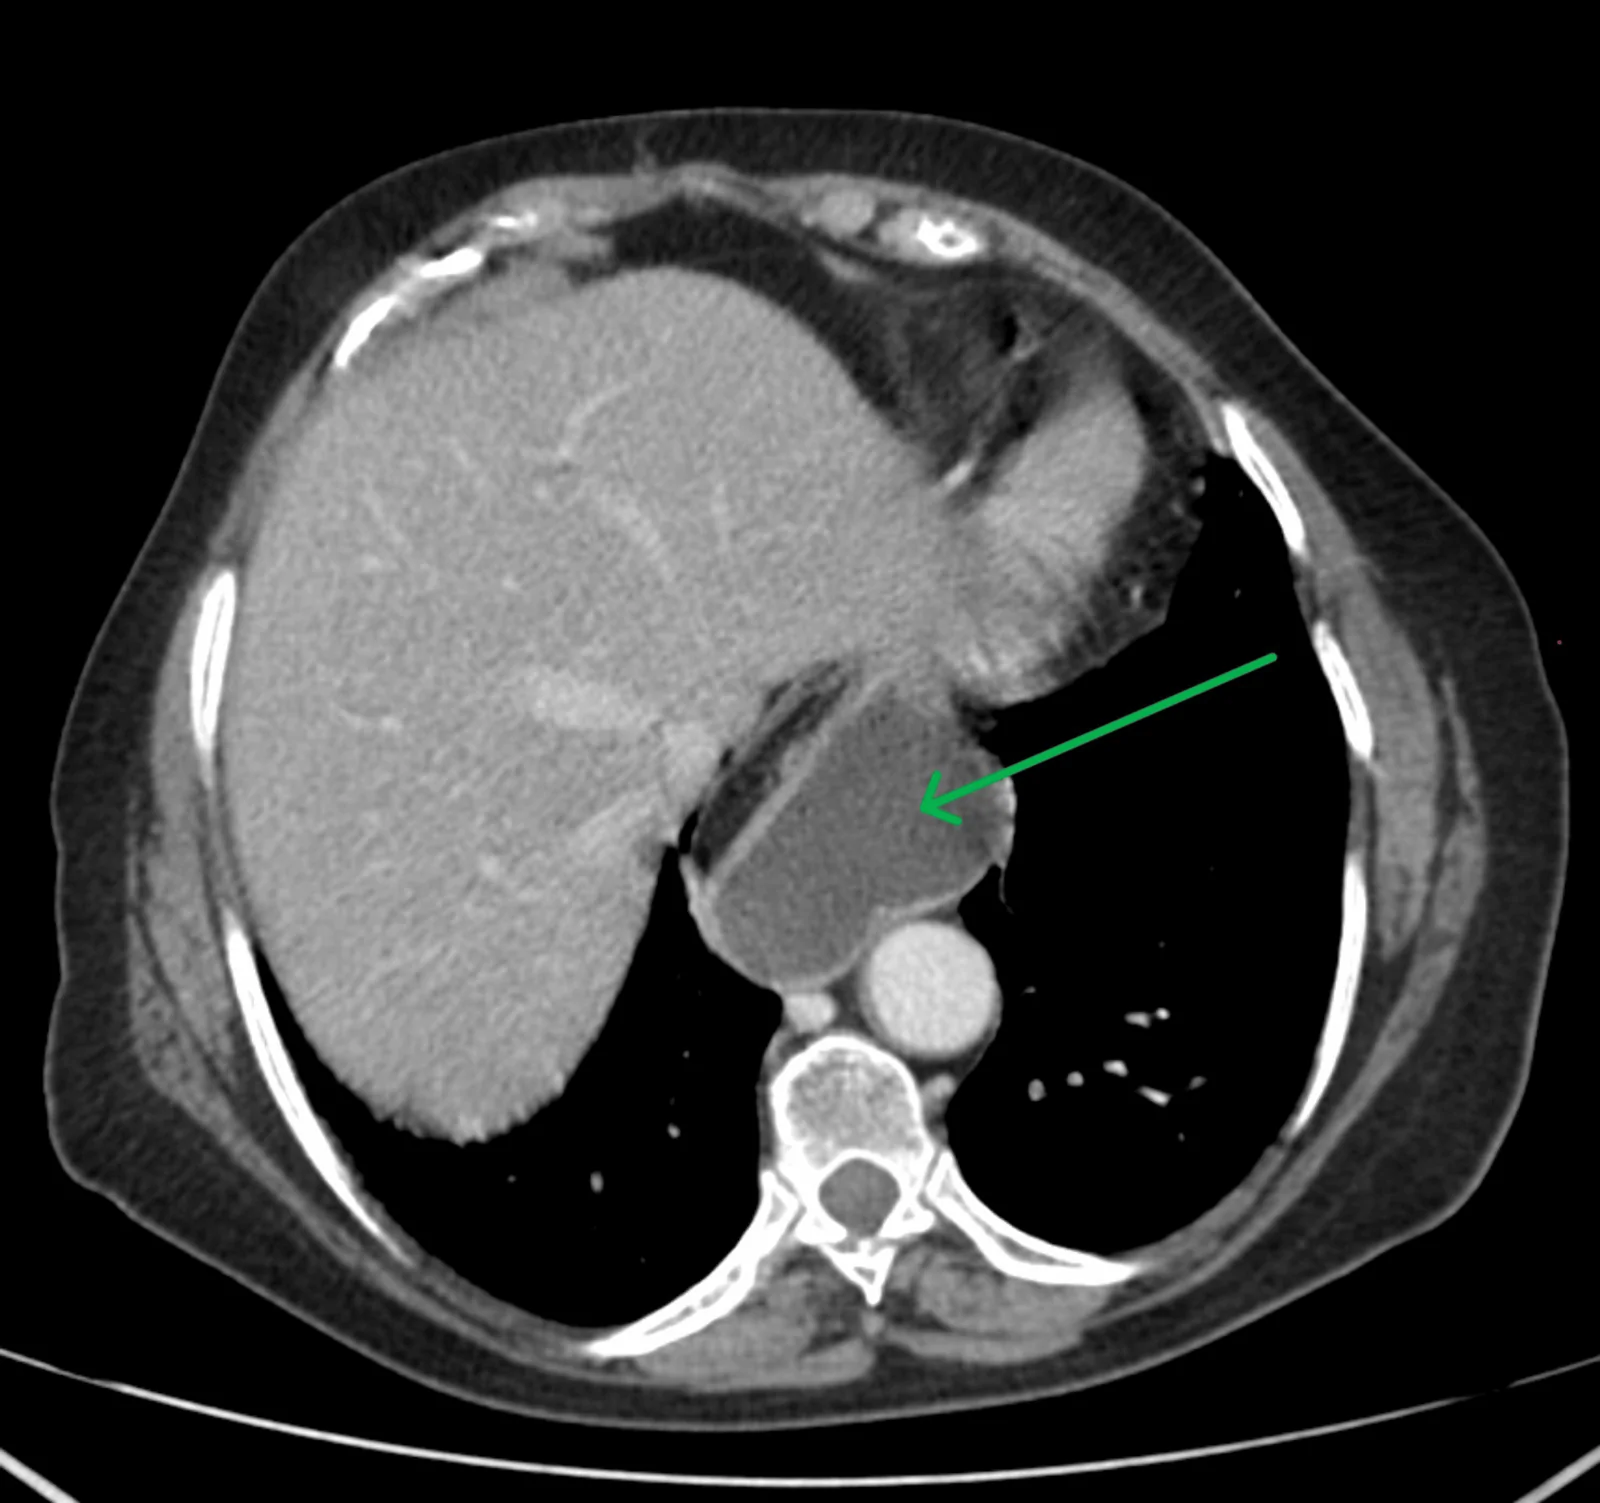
Contrast-enhanced thoracoabdominopelvic computed tomography showing esophageal dilatation (green arrow).
